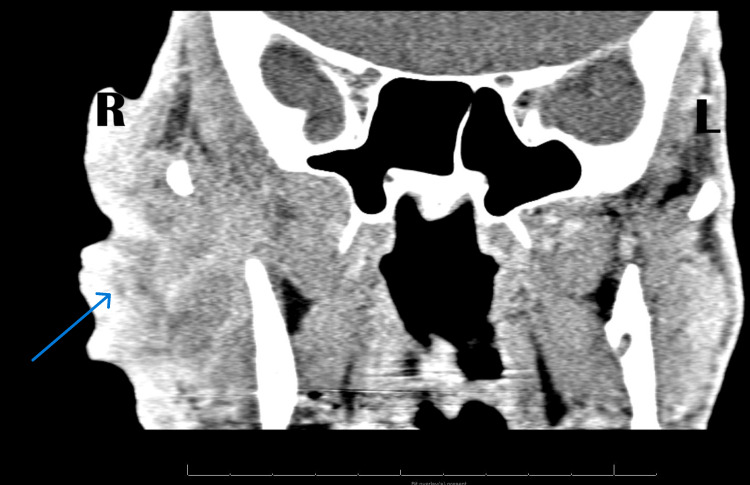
CT of the temporal bone with contrast in axial view showing deep tissue involvement on the right side.
